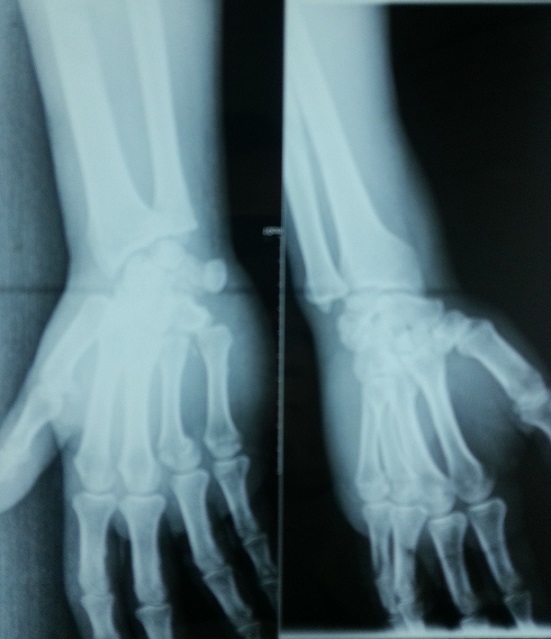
Les radiographies de face et de profil de la main droite objectivaient une luxation carpo-métacarpienne palmaire, associée à une fracture du col du quatrième métacarpe et une fracture de la base du premier phalange du cinquième doigt
